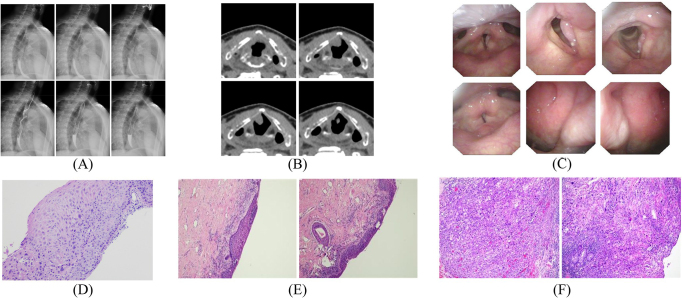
Examples of different image types used in laryngeal cancer assessment. (A) X-ray, (B) CT scan, (C) laryngoscopy image, (D) pre-operative, (E) intra-operative, and (F) post-operative pathology images.
